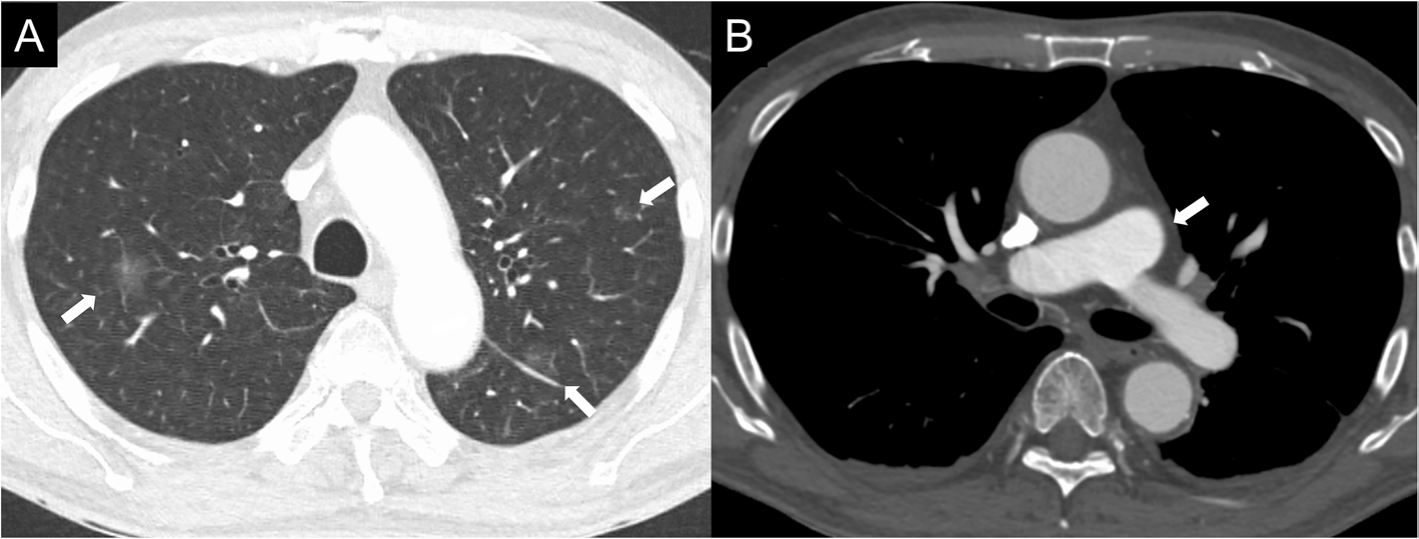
Chest computed tomography images. A Chest computed tomography (CT) shows patchy ground-glass opacities predominantly in both upper lobes of the bilateral lungs (arrows). B The pulmonary artery phase of the CT shows no dilatation of the main pulmonary artery trunk diameter and no contrast defects within the pulmonary arteries (arrow)
